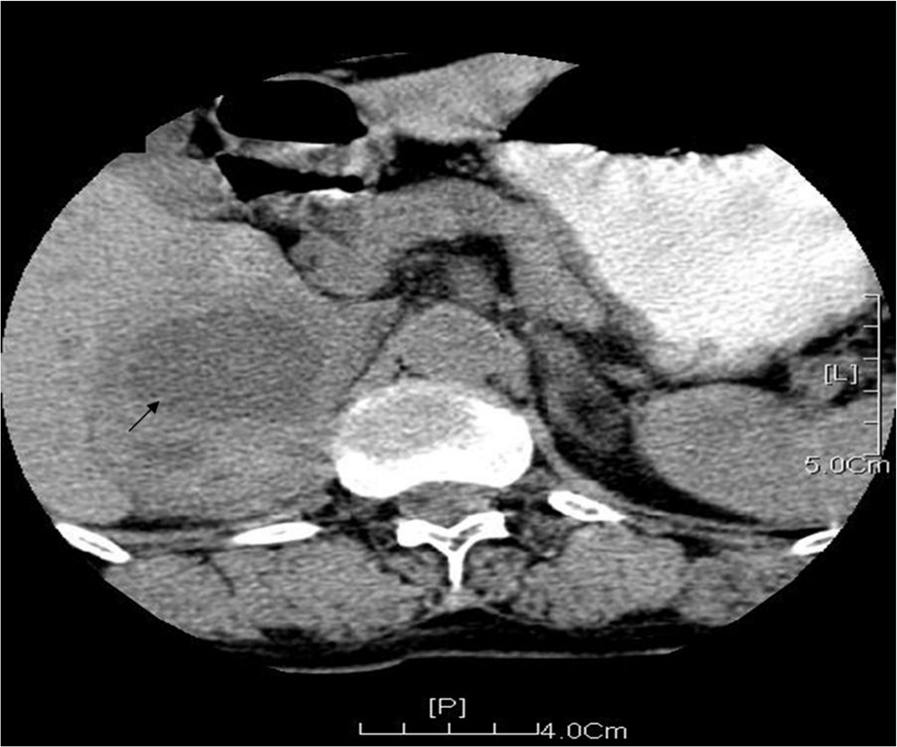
Plain CT scanning of the adrenal gland shows a uneven mass (Black arrow, about 6.2 × 6.7 × 7.8 cm) with intact capsule and punctate calcifications located at the right adrenal gland, which shares a unclear boundary with margo interior hepatics.
